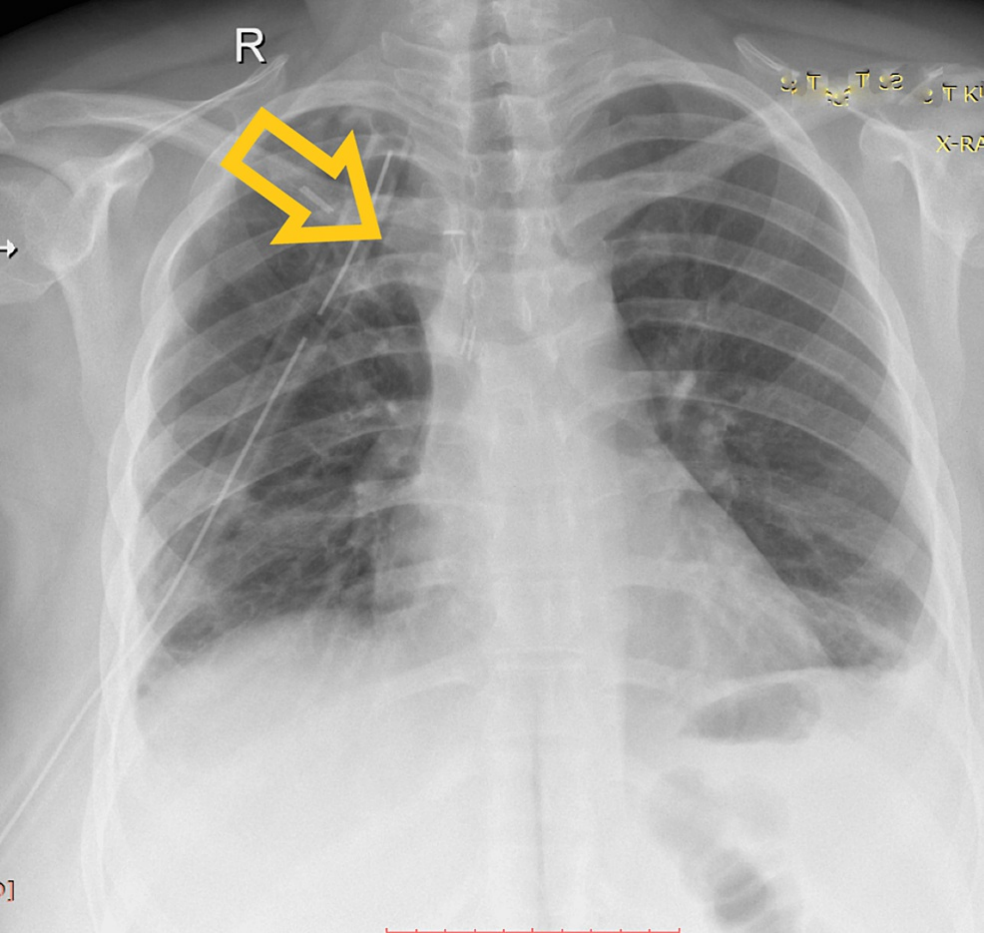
Postoperative chest X-ray The image shows an interval decrease in right-sided pleural effusion. The trachea is back in the midline as well as other mediastinal contents. The right-sided chest tube is in situ.
